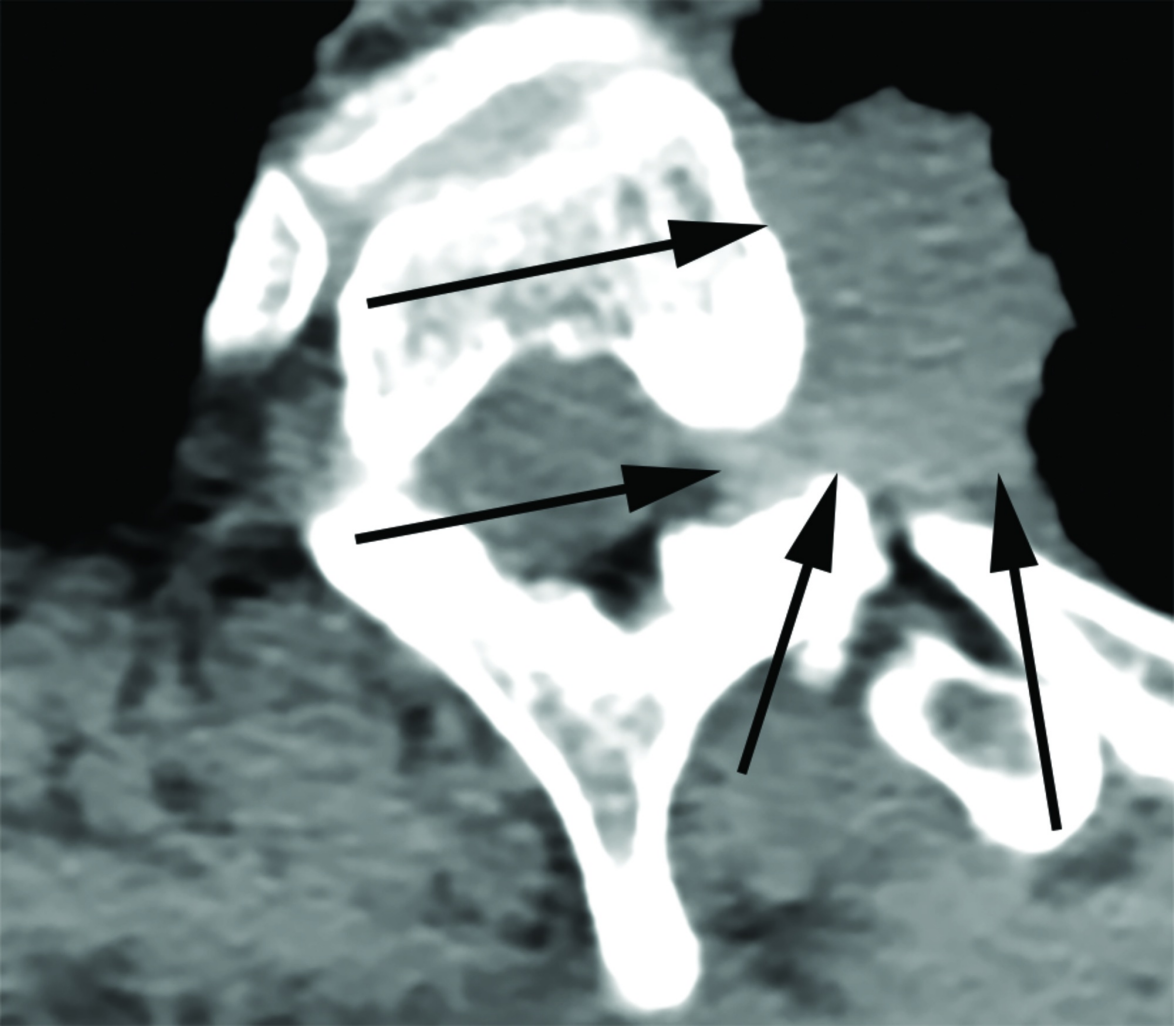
Intraoperative Axial CT of the T2 Vertebra Axial computed tomography (CT) image taken of the T2 vertebra with the O-Arm for anticipated osteotomy. Arrows delineate the tumor mass. O-Arm: Medtronic Corporation, Minneapolis, Minnesota, US
